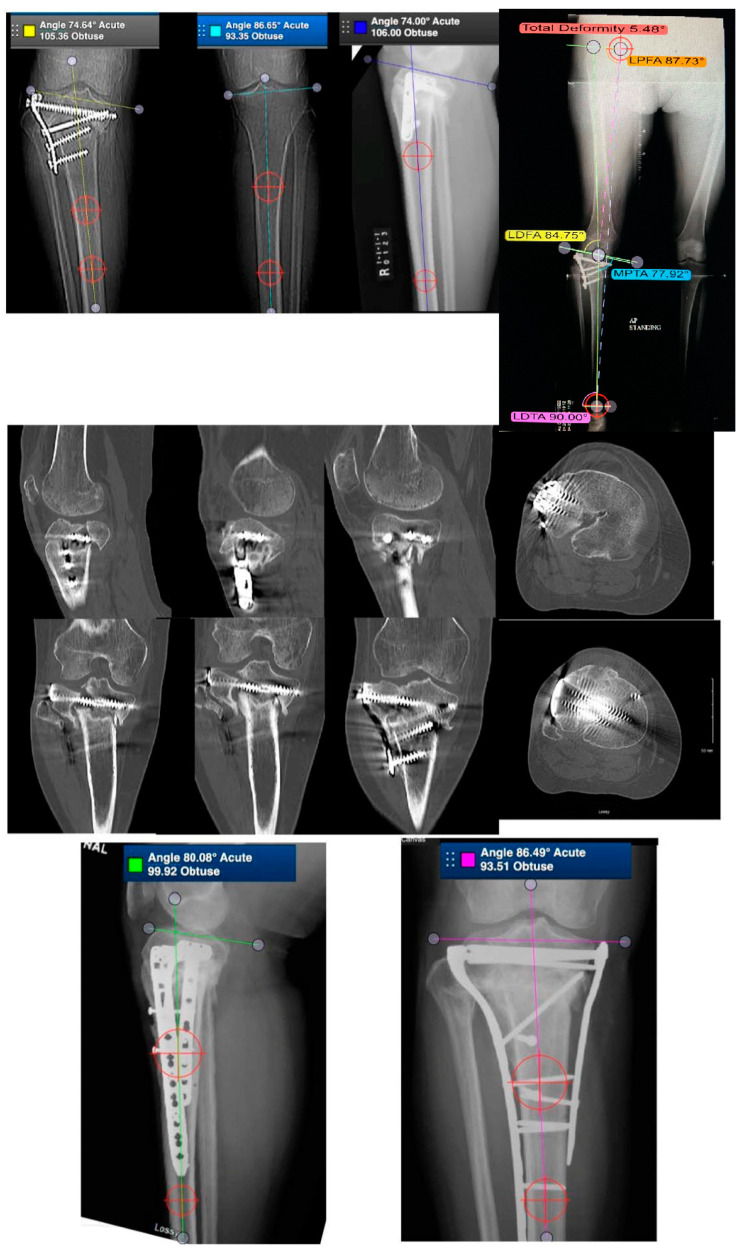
Case of a 54-year-old female with intra-articular malunion of the proximal tibia with 5 mm of lateral depression and 13 degrees of varus deformity (first row of images). CT scan is shown in the second row of images. Patient was treated with a lateral parapatellar approach with a lateral tibial tubercle osteotomy and a trap door osteotomy. The depressed fragments were elevated followed by an opening-wedge tibial osteotomy. Medial and lateral plates were applied to hold the reduction (third row of images).
